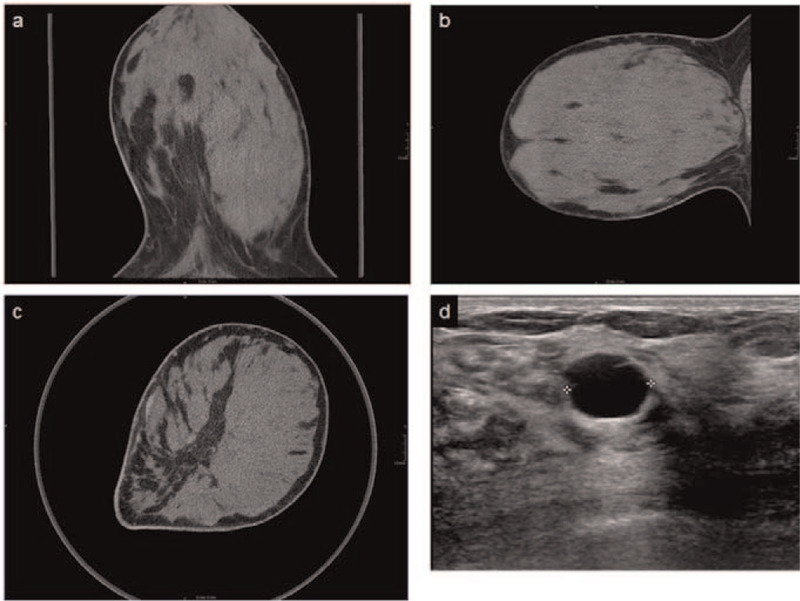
BCT slices axial (A), coronal (B) and sagittal (C) plane and corresponding ultrasound (D). Sonographic proven cyst (12 mm) not visible in BCT in a patient with very high BCTD without fatty septae in the densest parenchymal areas.
